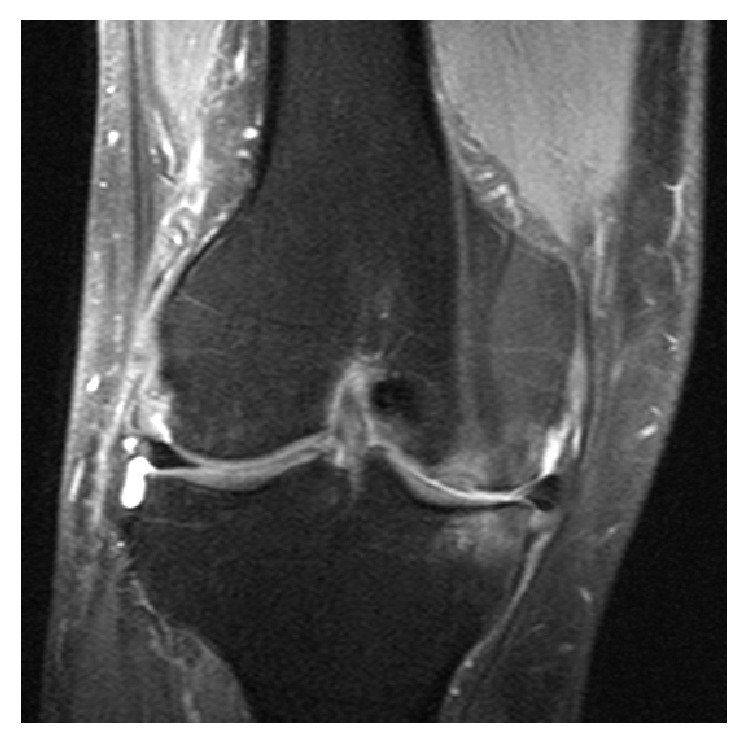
MRI of the knee: inflammatory changes with marked effusion and synovitis as well as subchondral edema at the medial tibia and the medial femur.
